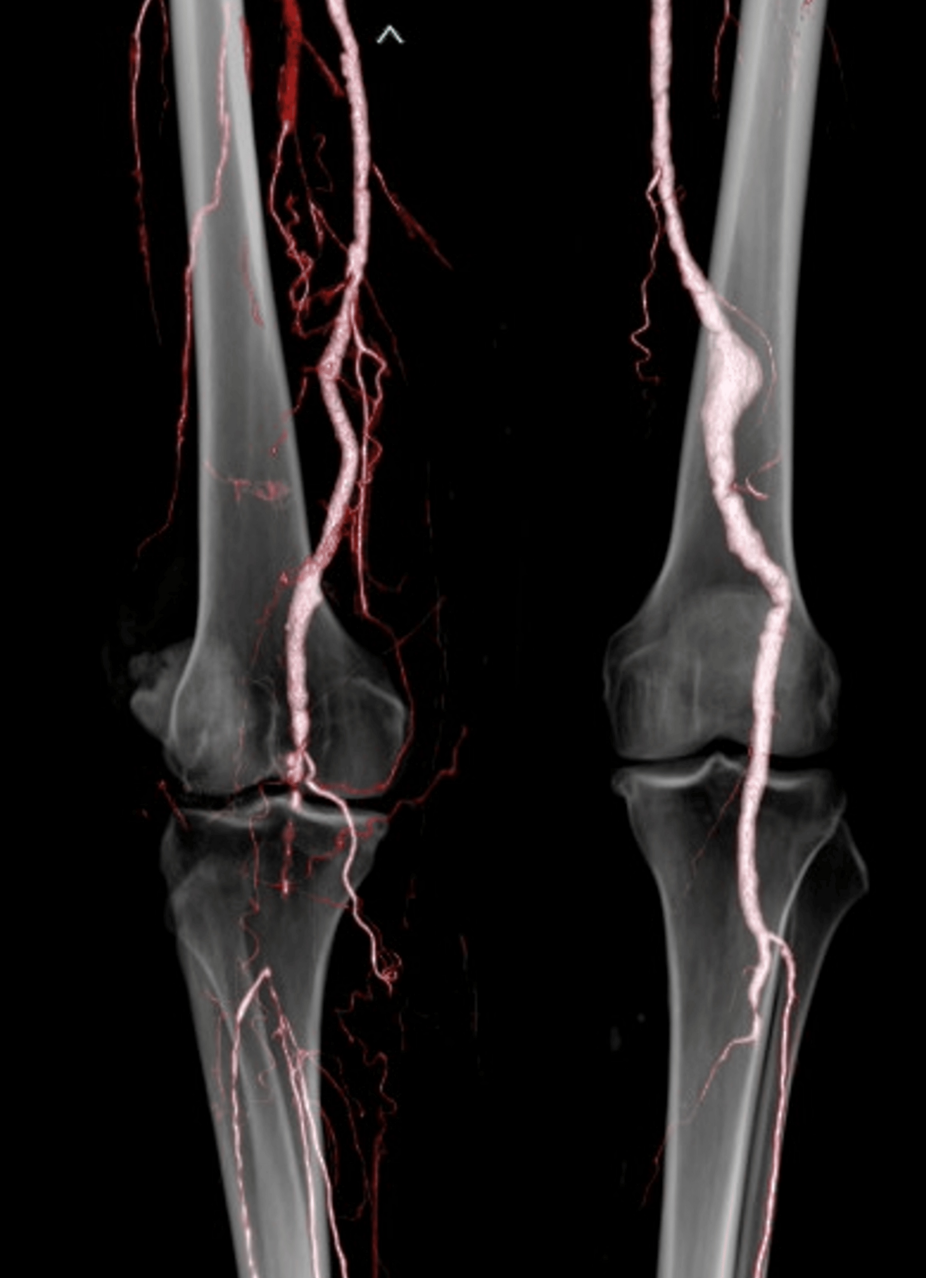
Postoperative computed tomography. Postoperative 3D enhanced computed tomography showed good blood flow in the popliteal artery, with sufficient flow observed below the knee.
